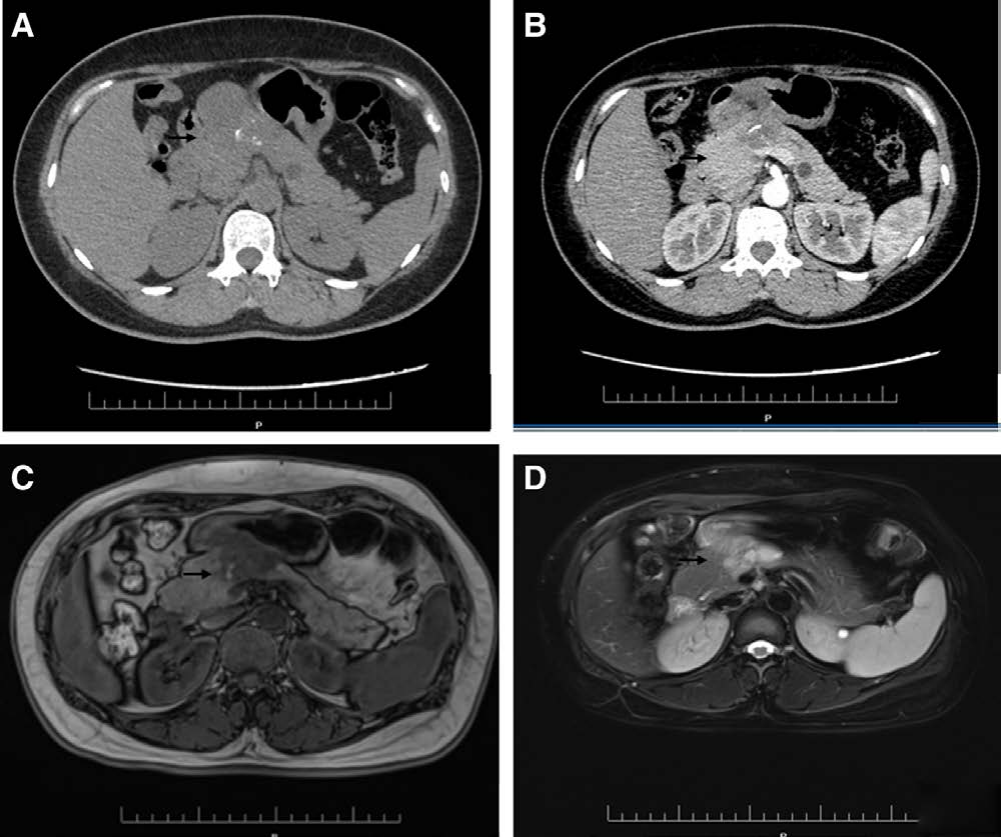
(A) Computed tomography scan of the whole abdomen showing that the shape and size of the pancreas are normal. (B) A mass of soft tissue density shadow is observed in the body of the pancreas; it has clear boundary, the size was 53 mm × 44 mm, the density is uneven, and calcification is observed. The CT value of the solid component was 32 HU. (C) Magnetic resonance imaging shows an abnormal mass of low signal intensity on T1WI and high signal intensity on T2WI in the body of the pancreas, and the lesion size is approximately 54 mm × 52 mm. The signal intensity of the lesion is uneven, and small nodular high signal intensity on T1WI is observed. (D) The lesion shows heterogeneous enhancement on contrast-enhanced scan. CT = computed tomography.
